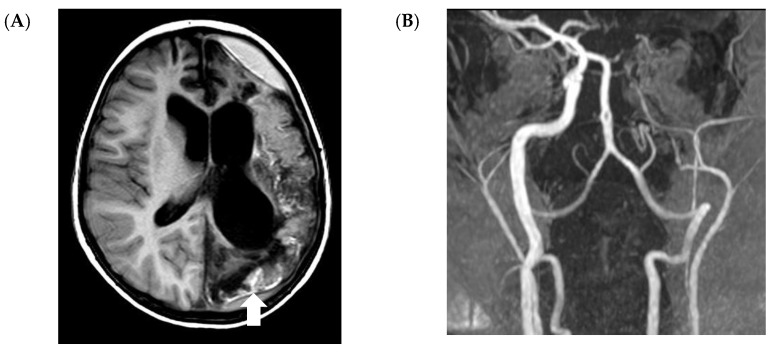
Vascular involvement in a 5-year-old boy with acute lymphoblastic leukemia (ALL) and probable central nervous system invasive mold infection. The patient is alive with residual right-sided hemiparesis. Magnetic resonance imaging (MRI) shows a large hemispheric infarction on the left side and cortical necrosis (arrow) on T1-W images without contrast (A). Time-of-flight magnetic resonance angiography (TOF-MRA) (B) shows only weak flow of the left internal cerebral artery.
